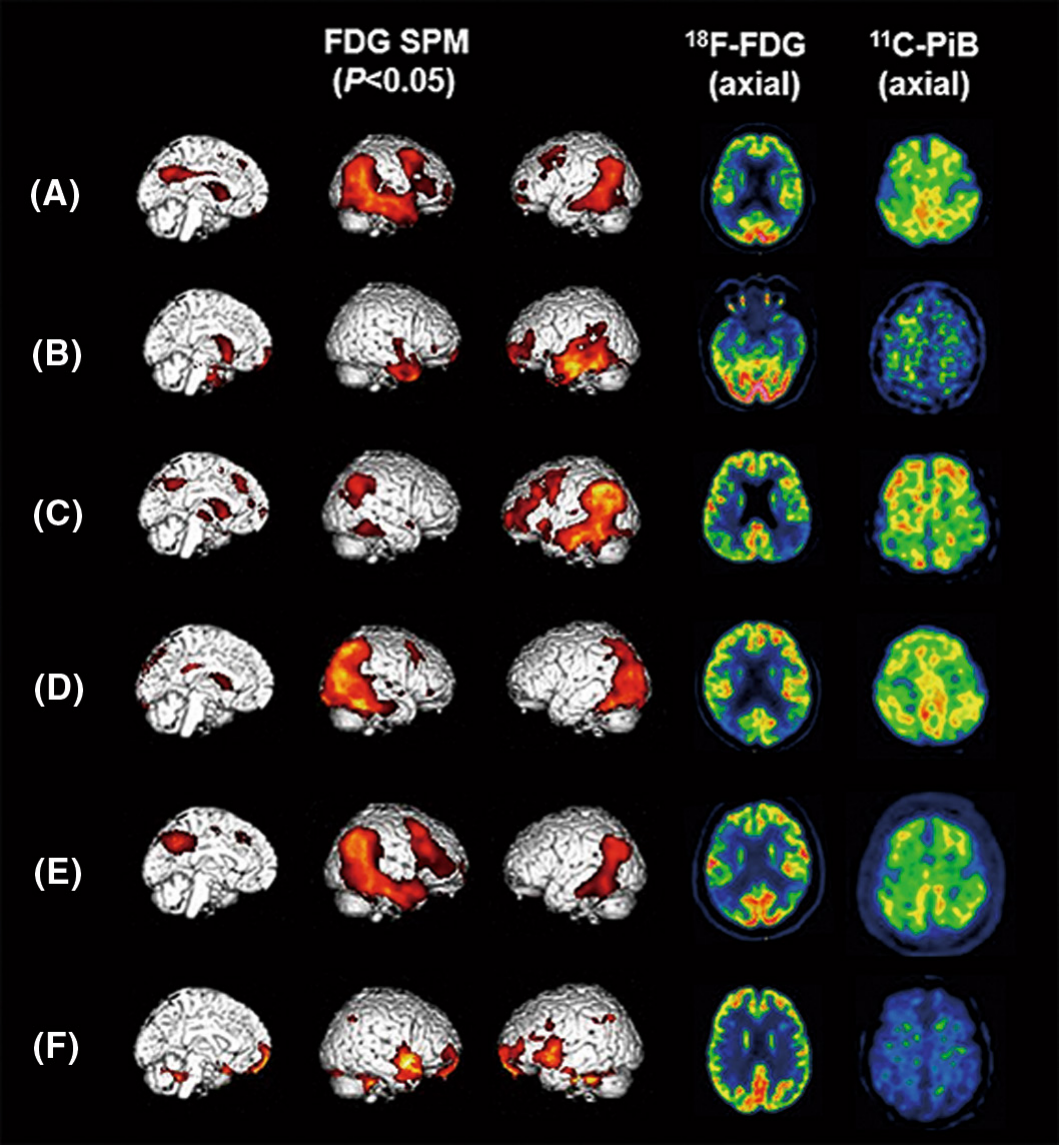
FDG‐PET and PiB‐PET images of six representative participants. (A) An AD patient with an “AD‐typical pattern” on the FDG‐PET image featuring predominant hypometabolism in the temporoparietal cortex and posterior cingulate cortex and with a positive PiB‐PET result. (B) A patient with a semantic variant of PPA due to FTLD who was initially diagnosed with AD before PET examination showed hypometabolism in the anterior temporal lobe and prefrontal lobes (“non‐AD pattern”) on the FDG image and had negative PiB‐PET results. (C) A patient with logopenic variant of AD who was initially diagnosed with a nonfluent variant of PPA before PET examination showed an “AD‐typical pattern” but left predominant hypometabolism on the FDG image and had positive PiB results. (D) A patient with posterior variant of AD who was initially diagnosed with DLB before PET examination showed hypometabolism in the occipital lobes and posterior parietal lobes on the FDG image and had positive PiB results. (E) An MCI patient converted to AD dementia 14 months after PET examination and showed an “AD‐typical pattern” on the FDG image with positive PiB results. (F) An MCI patient remained stable 17 months after PET examination and showed a “non‐AD pattern” with nonspecific hypometabolism in a few temporal and frontal areas on the FDG image and had negative PiB results. AD, Alzheimer's disease; DLB, dementia with Lewy bodies; FDG, fluorodeoxyglucose; MCI, mild cognitive impairment; PET, positron emission tomography; PiB, Pittsburgh compound B; PPA, primary progressive.
